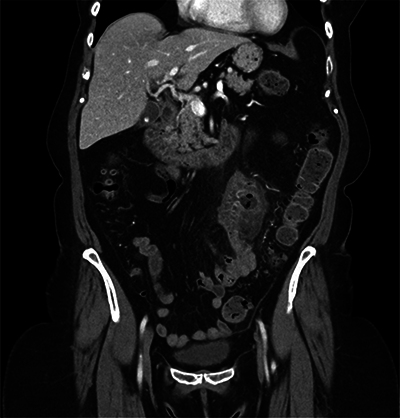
Coronal contrast-enhanced CT scan highlighting uncomplicated jejunal diverticulitis.
